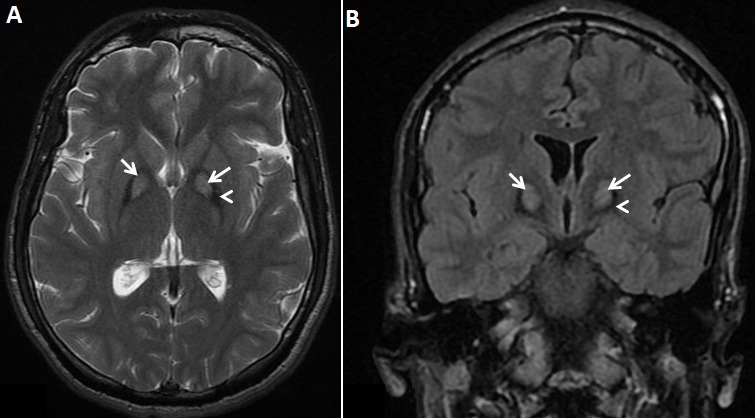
(a) : IRM cérébrale, coupe axiale en séquence T2 objectivant un hypersignal bilatéral et symétrique des globi pallidi (flèche), bordé d'un hyposignal net (tête de flèche) donnant un aspect caractéristique en œil de tigre. (b) : IRM cérébrale, coupe coronale en séquence FLAIR objectivant l'anomalie de signal des globi pallidi se présentant en hypersignal central (flèche) en rapport les lésions neuro-axonales, avec un liseré périphérique en hyposignal (tête de flèche) en rapport avec les dépôts de fer
